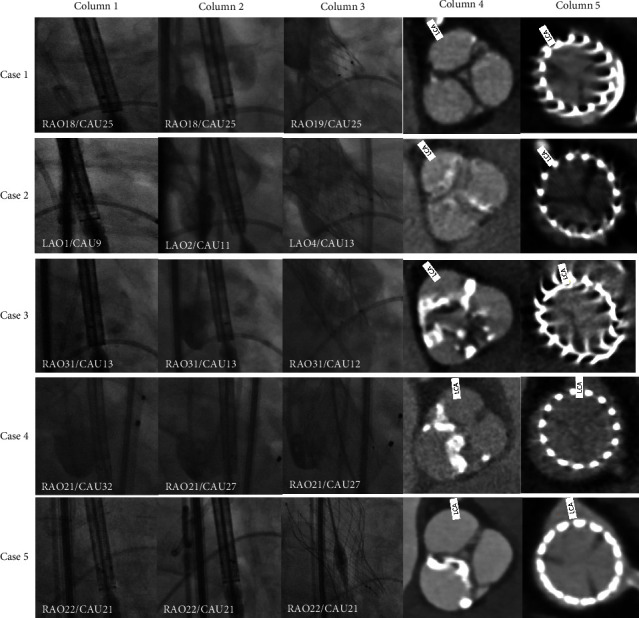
Neo-commissure alignment by manipulating the delivery system. Column 1: angiograms when the THV crossed the aortic annulus at the first time. The centre markers were close to or overlapped with the left markers. Column 2: angiograms after the delivery system were withdrawn to the descending aorta and readvanced to the aortic annulus. The centre markers were close to or overlapped with the right markers. Column 3: angiograms after the THV were deployed. Column 4: transverse aortic sinus CT images before TAVR. Column 5: transverse aortic sinus CT images after TAVR. THV = transcatheter heart valve; CT = computed tomography; TAVR = transcatheter aortic valve replacement; RAO = right anterior oblique; LAO = left anterior oblique; CAU = caudal; and LCA = left coronary artery.
